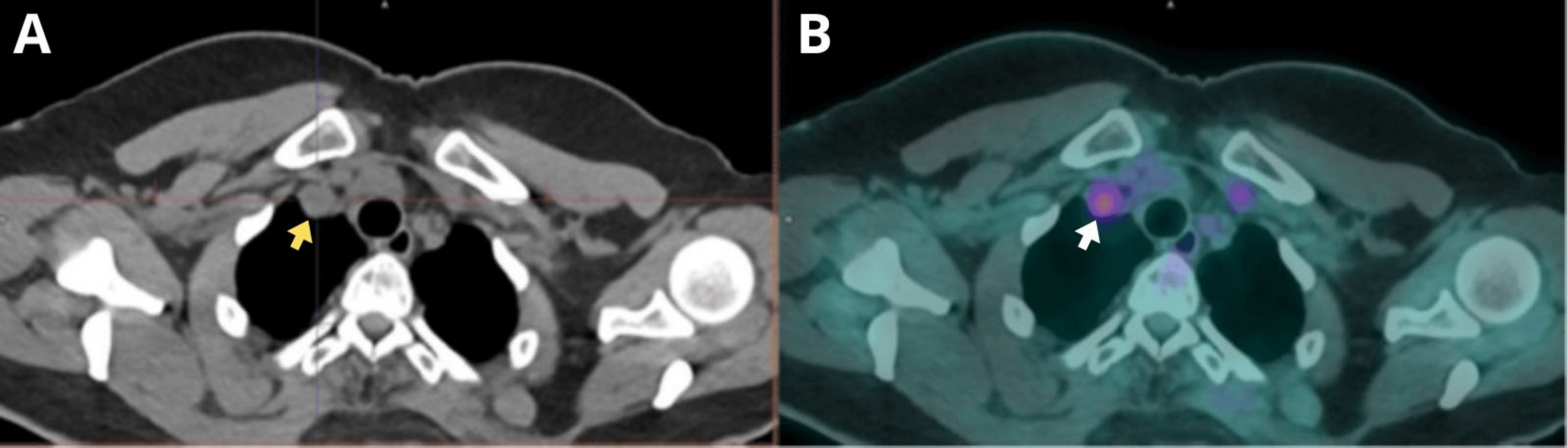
A) Axial CT scan showing a lymph node in the right level IV cervical region, measuring 1.1 cm (yellow arrow). B) Axial fused PET/CT image demonstrating hypermetabolism in the cervical lymph node, SUVmax of 4.1 (white arrow). CT: computed tomography; PET/CT: positron emission tomography/computed tomography
